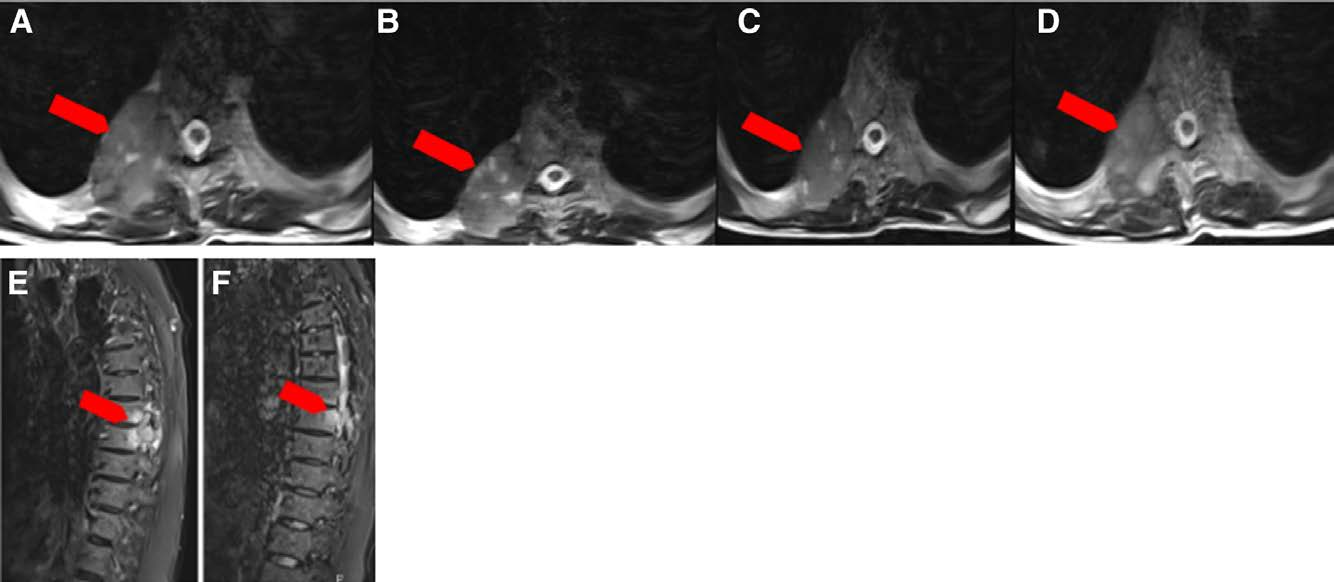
Thoracic MRI showed abnormal bone signals (red arrows) in the right 8th and 9th posterior ribs, T8 and T9 vertebral bodies, and right adnexa, with the formation of a soft tissue (red arrows) mass in the surrounding area side. (A–D) Axial views, (E, F) sagittal views. MRI = magnetic resonance imaging.
